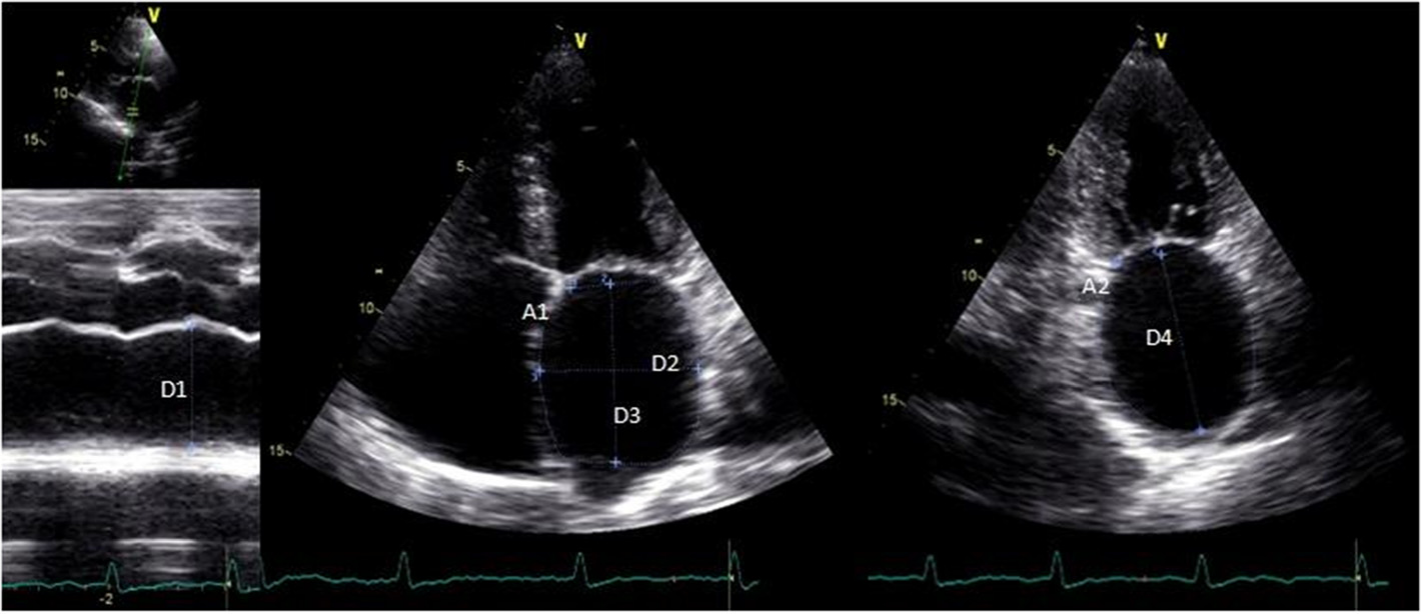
Echocardiographic parameters used to calculate the left atrial volumes. Legend: A1 - LA area in 4C view; A2 - LA area in 2C view; D1 - LA AP diameter; D2 - medial–lateral diameter; D3 - superior-inferior diameter in 4C view; D4 - superior-inferior diameter in 2C view.
